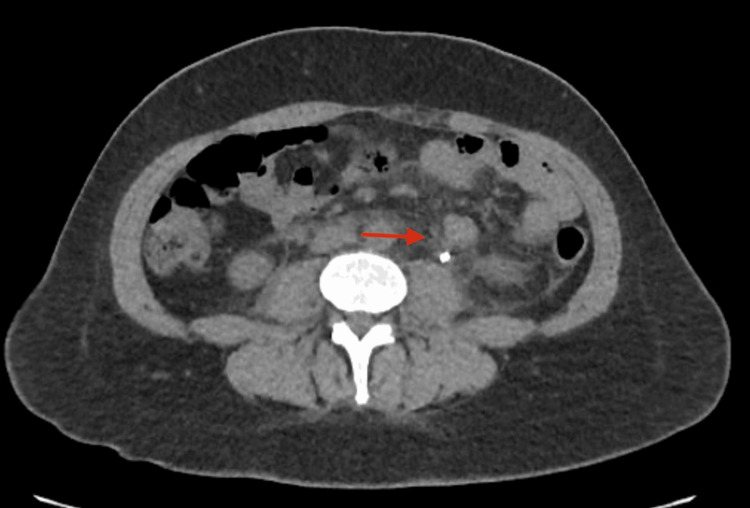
Axial CT abdomen and pelvis without contrast showing trace left perinephric fluid (red arrow) indicative of calyceal rupture
